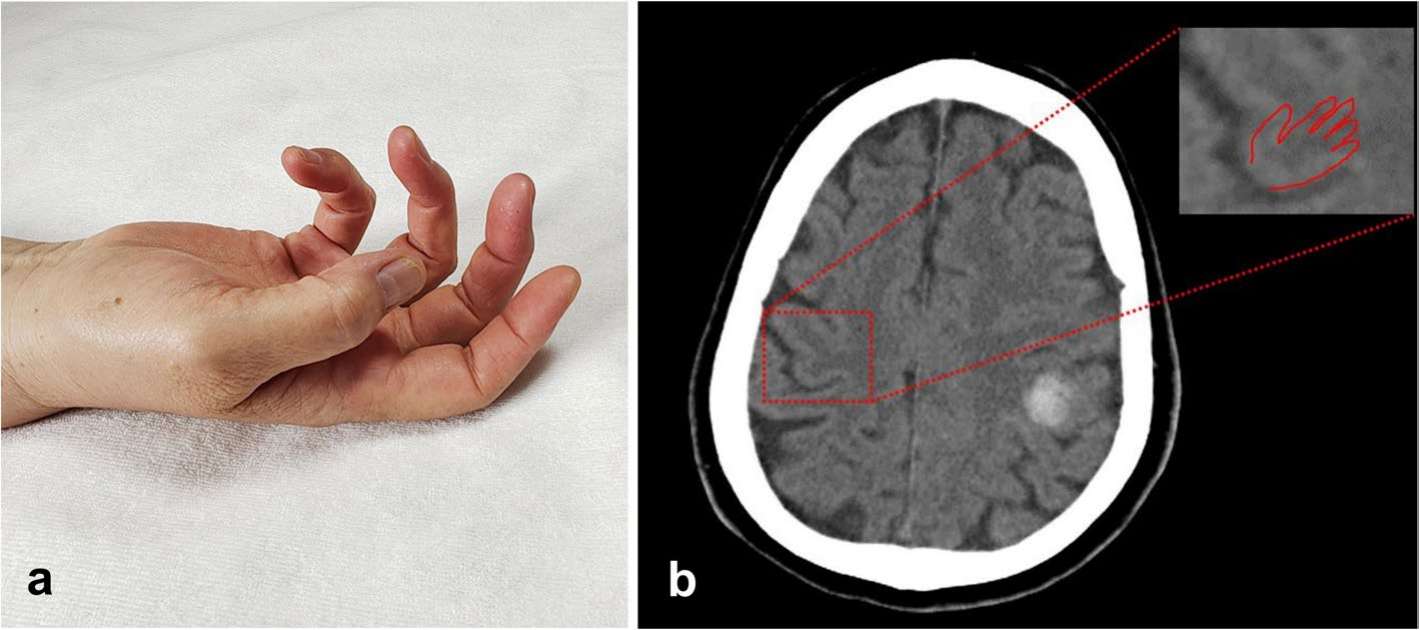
a 79-year-old man with a previous history of severe hypertension presented with acute isolated total hand weakness; b Cranial CT showed cerebral hemorrhage in the left knob area
